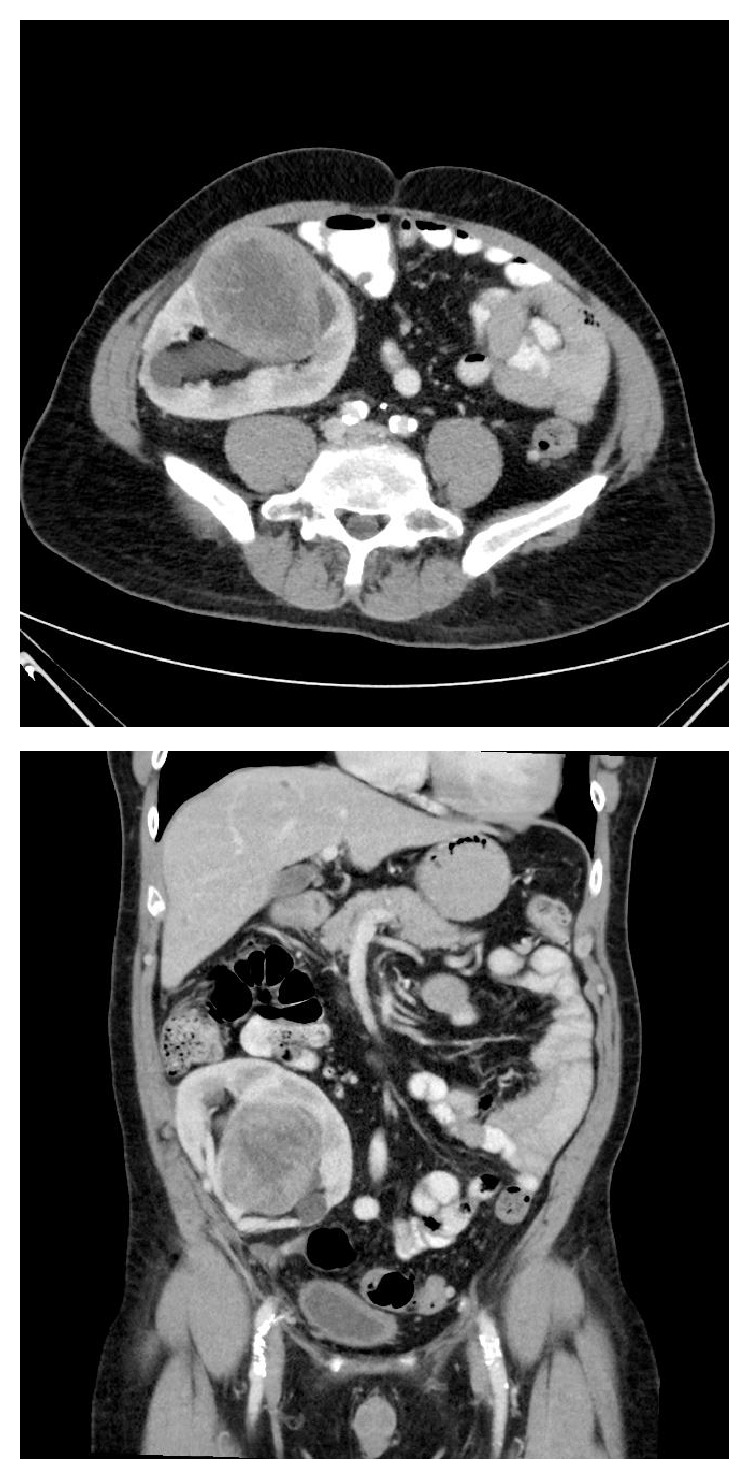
Axial and coronal contrast-enhanced computed tomographic images showing a heterogeneous, well circumscribed, soft tissue mass arising from the midpole of the renal allograft showing minor vascularity and less enhancement compared to surrounding parenchyma.
